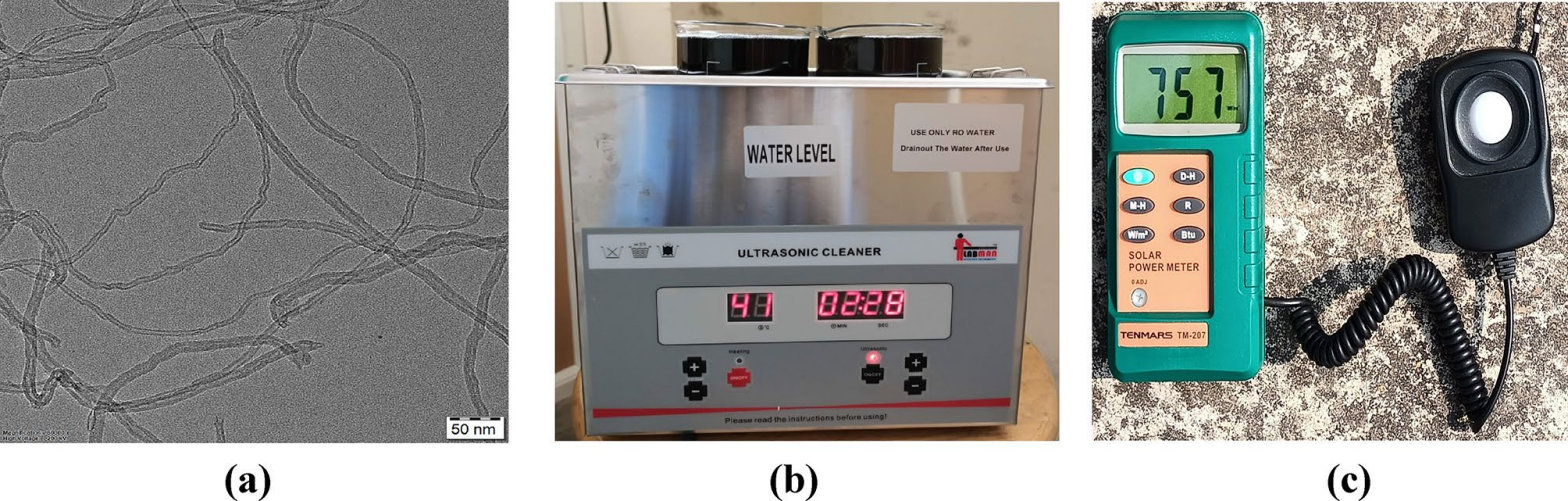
The characterization, instrument used for the preparation of nanofluid and measurement of solar radiation (a) MWCNT TEM image (b) Ultrasonic bath for nanofluid (c) solar power meter.
